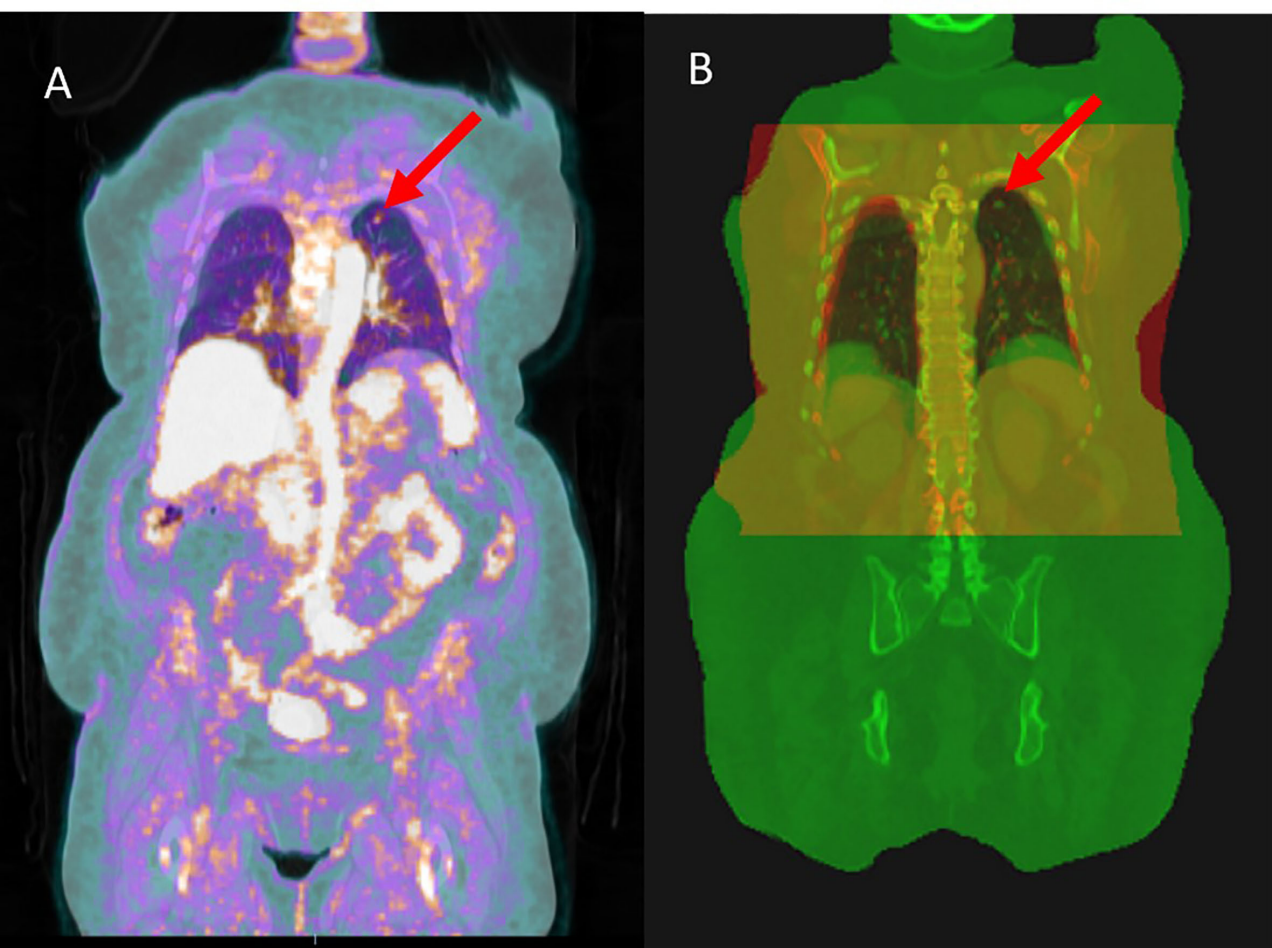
(A) PET scan overlaid on PET/CT scan, used for the initial diagnosis of the tumor (red arrow). The tumor is recognized by its high uptake of fluorine-18-fluorodeoxyglucose (F-FDG) (B) pCT scan (red) overlaid on the PET/CT scan (green), and the overlapping area is shown as yellow. One of the main differences between the two scans is the diaphragm level owing to the motion management technique.
